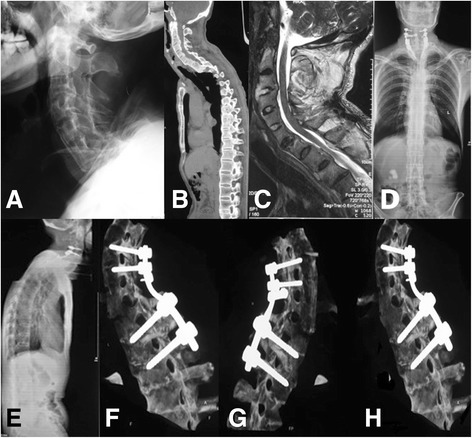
A 53-year-old man with ankylosing spondylitis and a complete fracture of C7 and partial dislocation. a Preoperative plain radiograph showing a displaced fracture together with an obvious gap in the anterior column at C7 because the rigid elevation of the shoulders prevents adequate imaging at the cervico-thoracic junction and so called bamboo spine resulting from ankylosing spondylitis; (b) and (c) CT with sagittal reconstruction and MR imaging showing an obvious fracture of C7. d and (e) Immediate antero-posterior and lateral radiographs showing posterior stabilization using pedicle screw fixation between C4 and T2. f and (g) Postoperative CT with sagittal reconstruction showing good screws placement in the cervical and thoracic pedicles, the cervicothoracic pre-existing kyphosis has been corrected. h Bone fusion has been confirmed by sagittal reconstruction CT after 6 months postoperatively
